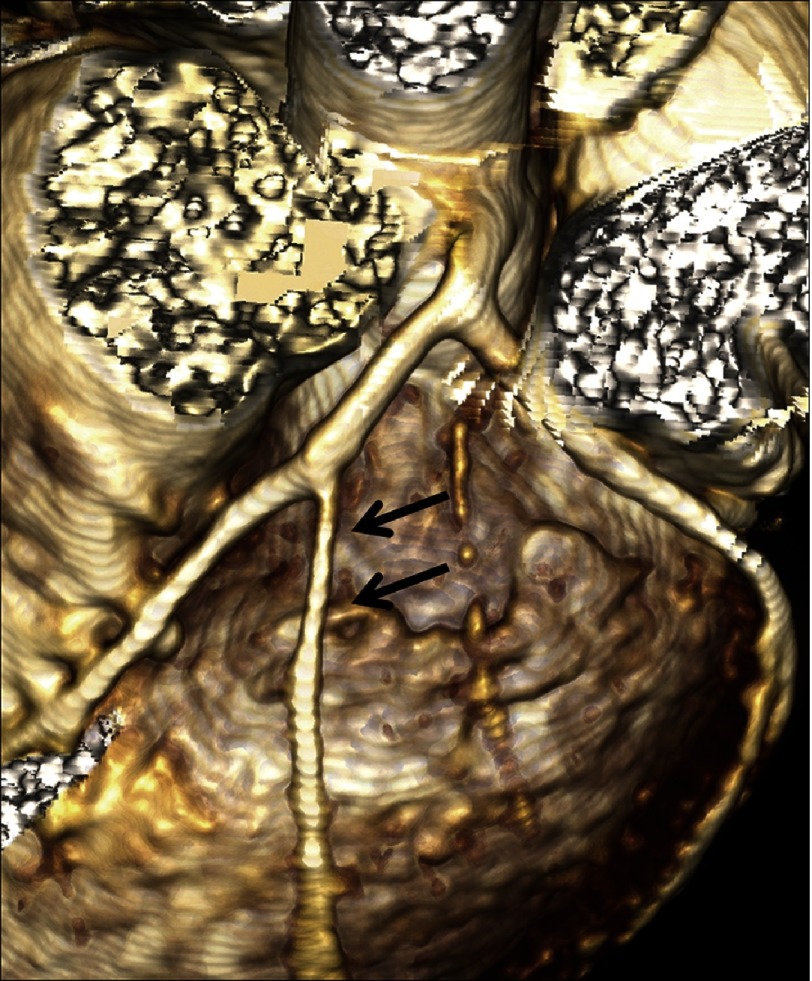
CTCA VRT 8 year female child in convalescent phase (5 years after diagnosis) show long segment stenosis of diagonal branch of LAD (arrows). 2D-transthoracic echocardiography has limitation to detect coronary artery abnormalities of coronary artery branches.
